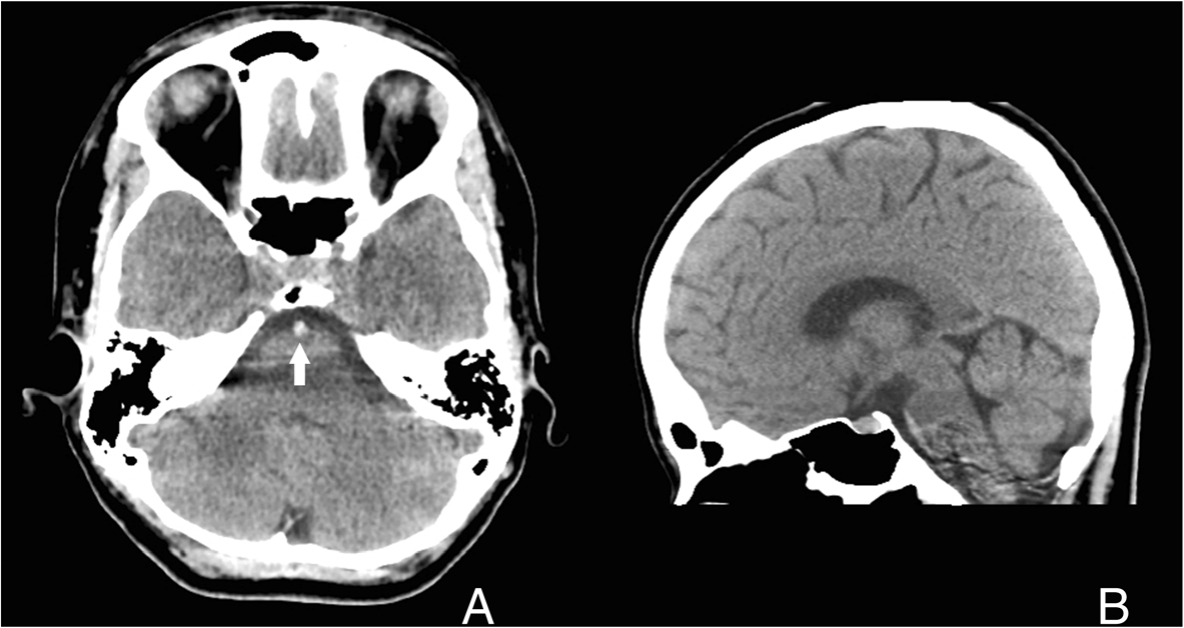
a Non-contrast CT (*axial view*) showing the hyperdense basilar artery sign (*arrow*). b Non-contrast CT (*sagittal view*) with the hyperdense basilar artery seen anterior to the pons
